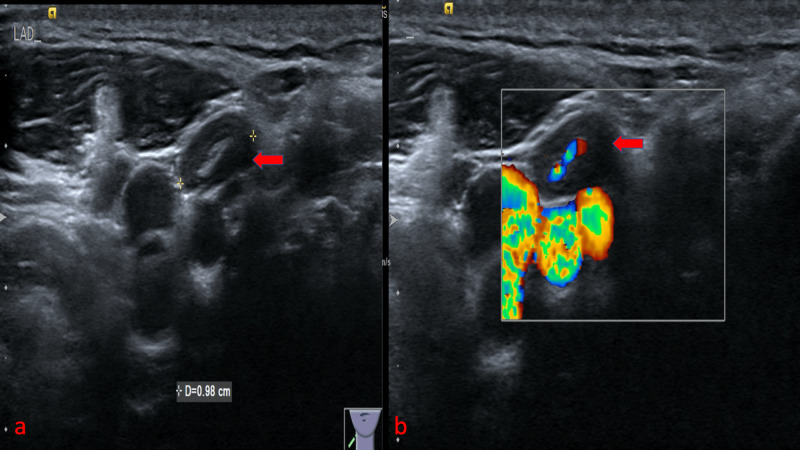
Imaging of normal cervical lymph node: (a) BMUS shows a lymph node with central fatty hilum (red arrow), (b) CDUS shows the hilar flow (red arrow) BMUS: B-mode ultrasound; CDUS: color doppler ultrasound
